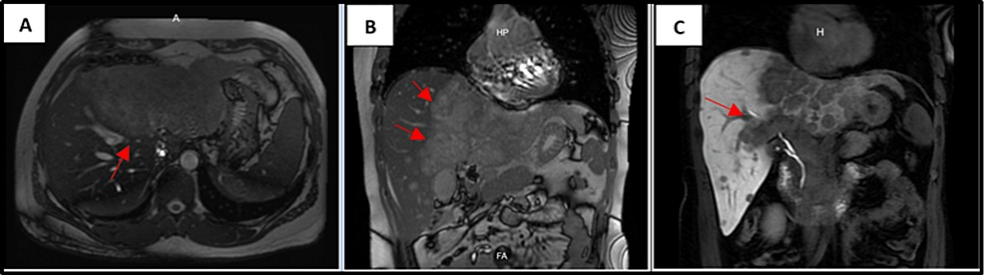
MRI demonstrated an irregular and heterogeneous mass (A). The mass showed multiple multisegmented nodular lesions in the right lobe (B). Biliary tree infiltration (C). MRI: magnetic resonance imaging
